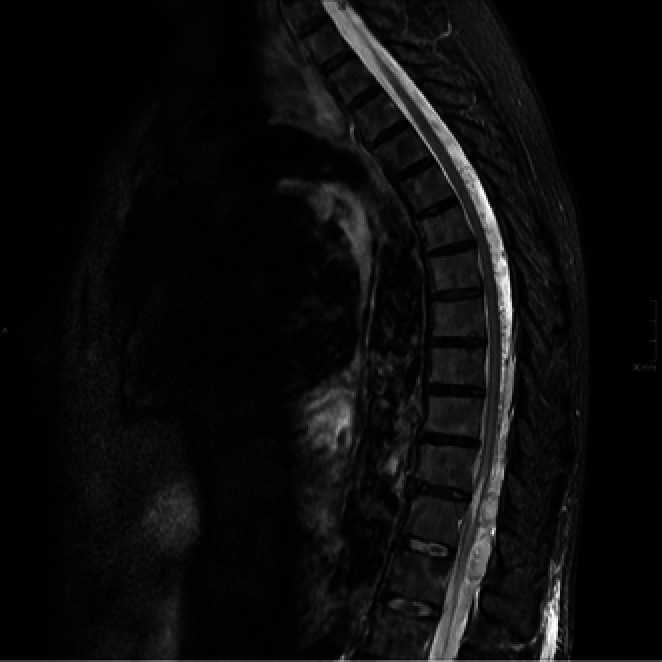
FIGURE 1 Sagittal STIR spinal MRI demonstrating an extensive hyperintense extradural collection consistent with an acute spinal epidural hematoma extending from T7 to L3, with maximal spinal cord compression and associated myelopathy at T11–T12.
